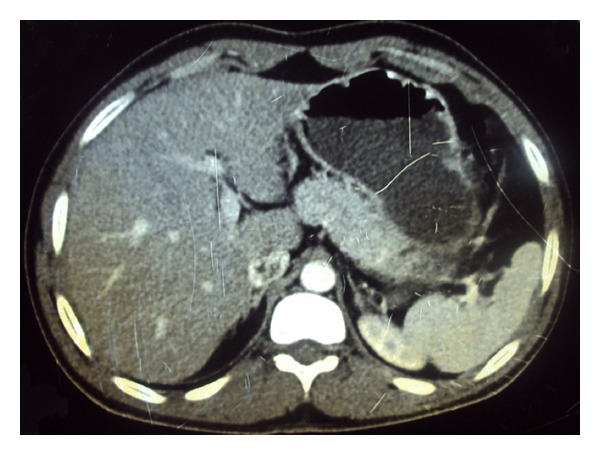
CT showing no involvement of the liver.
